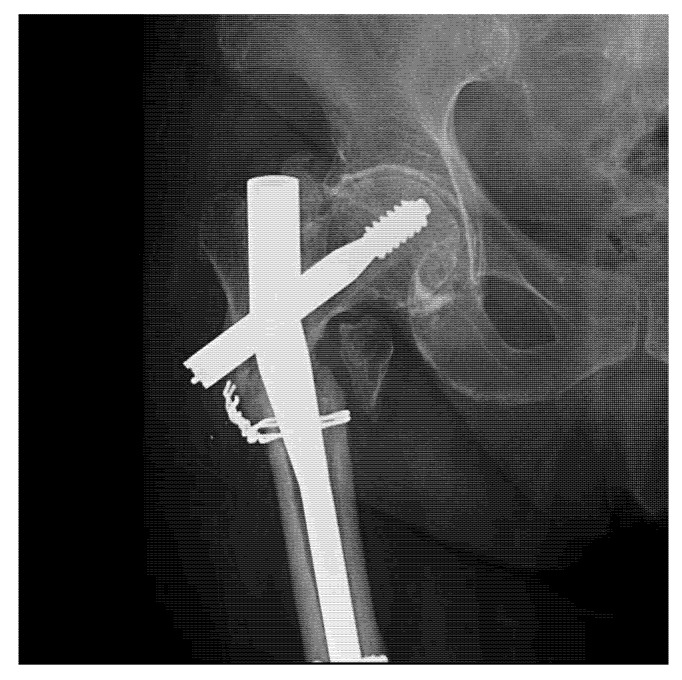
Radiography of a clinical case with failure of a Gamma Nail—72-year-old female patient.
